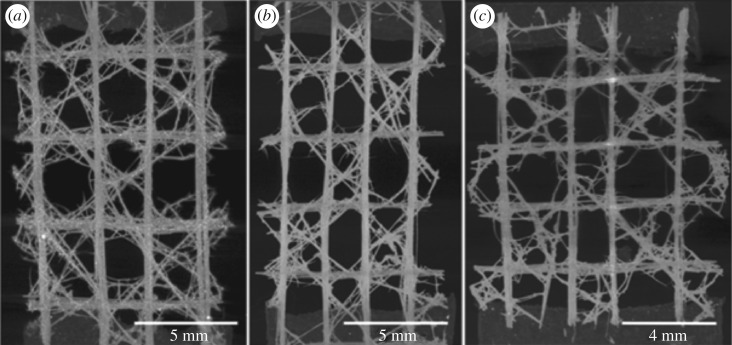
μ-CT maximum intensity projection images of the three different biological samples in *xz*-plane. (*a*) Closed Cell 1, (*b*) Closed Cell 2 and (*c*) Closed Cell 3.
